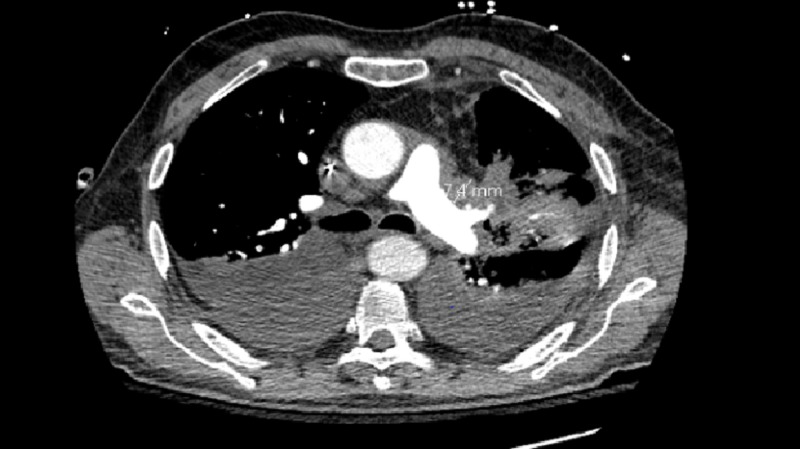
CT scan showing 7.4 mm mass in left lingula Bilateral pleural effusions, diffuse pleural thickening,and bilateral lung base air space opacity,soft tissue density in bilateral hilar region and bilateral bronchus wall thickening more prominent on the left, pleural effusion  given slight loculated appearance especially on the left side Presence of diffuse osteoblastic metastatic disease
